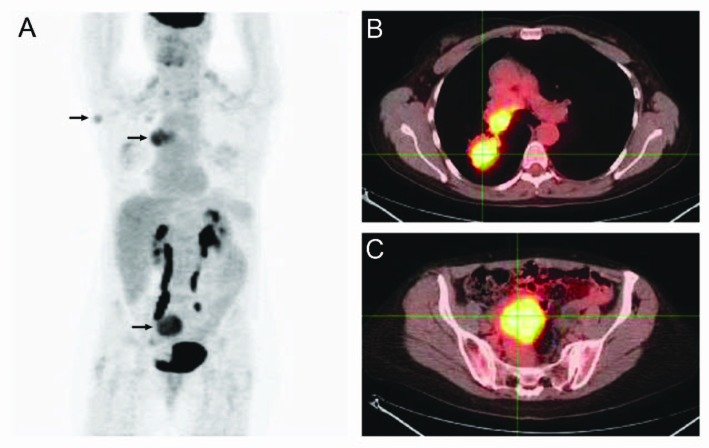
PET/CT study: (A) Whole body PET; (B) PET/CT overlay of chest; (C) PET/CT overly of pelvis. Arrows: hypermetabolic foci on PET.
